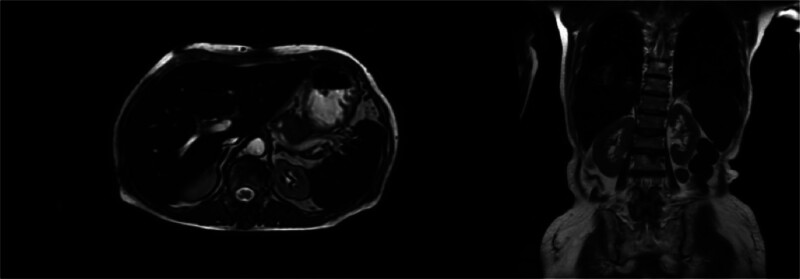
MRI examination results. MRI showing hypointense signals in the liver and spleen consistent with iron overload. MRI = magnetic resonance imaging.
